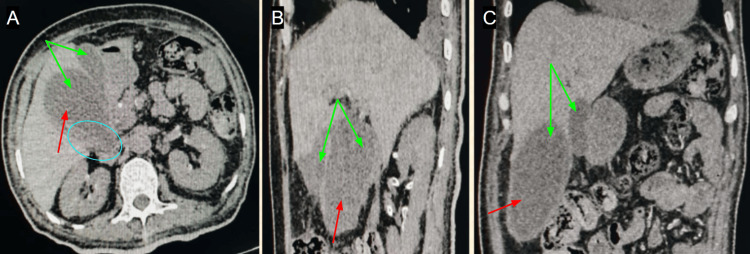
CT (A) axial, (B) sagittal, and (C) coronal reformatted images showing duplicated gallbladder with one of these appearing markedly distended (mucocele), showing dependent calculi. Green arrows: duplicated gallbladder; red arrows: distended gallbladder; and cyan circle: calculi
